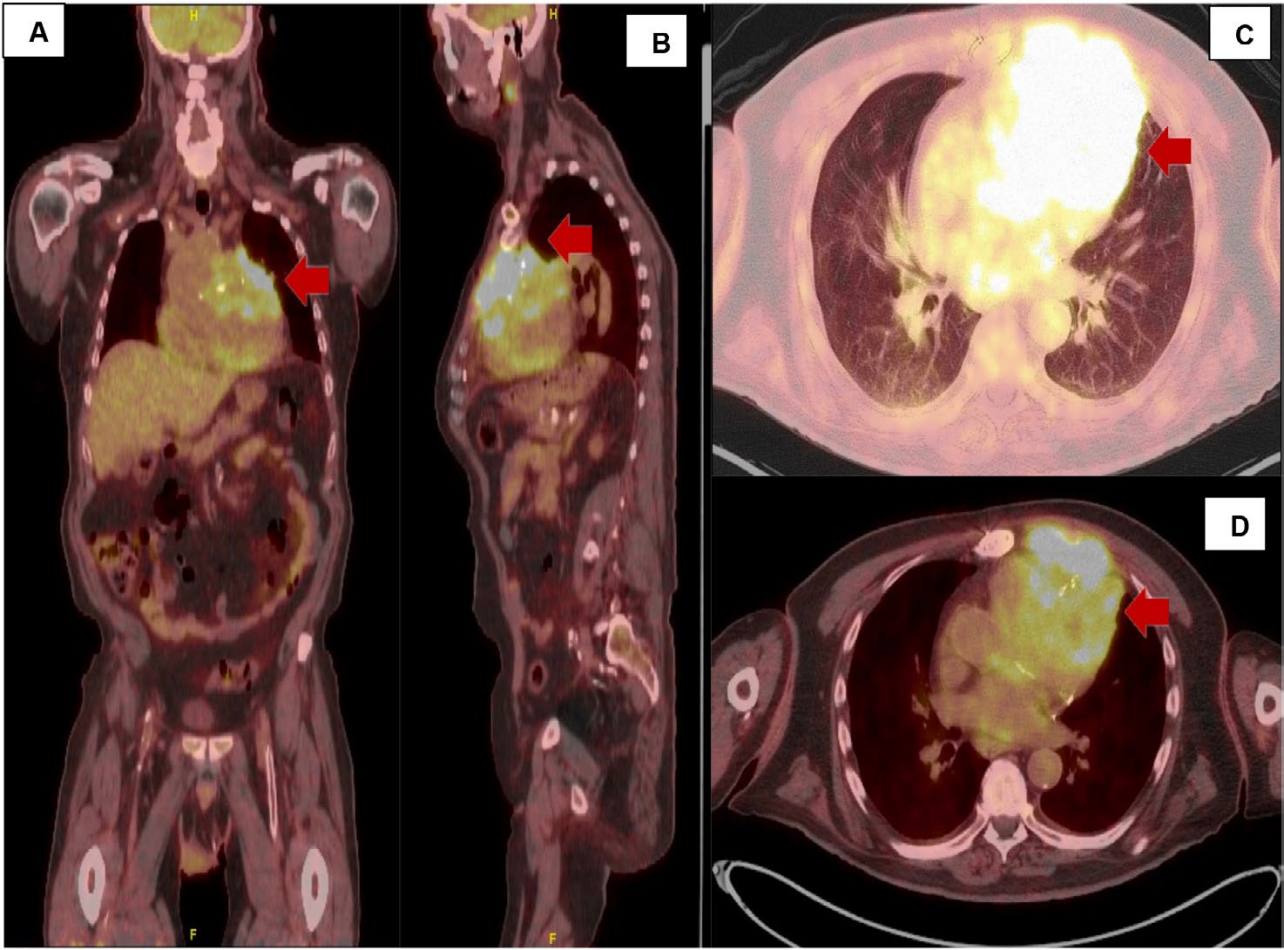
(A–D) PET CT confirms the presence of anterior thoracic mass invading the cardiac structure (red arrow).
